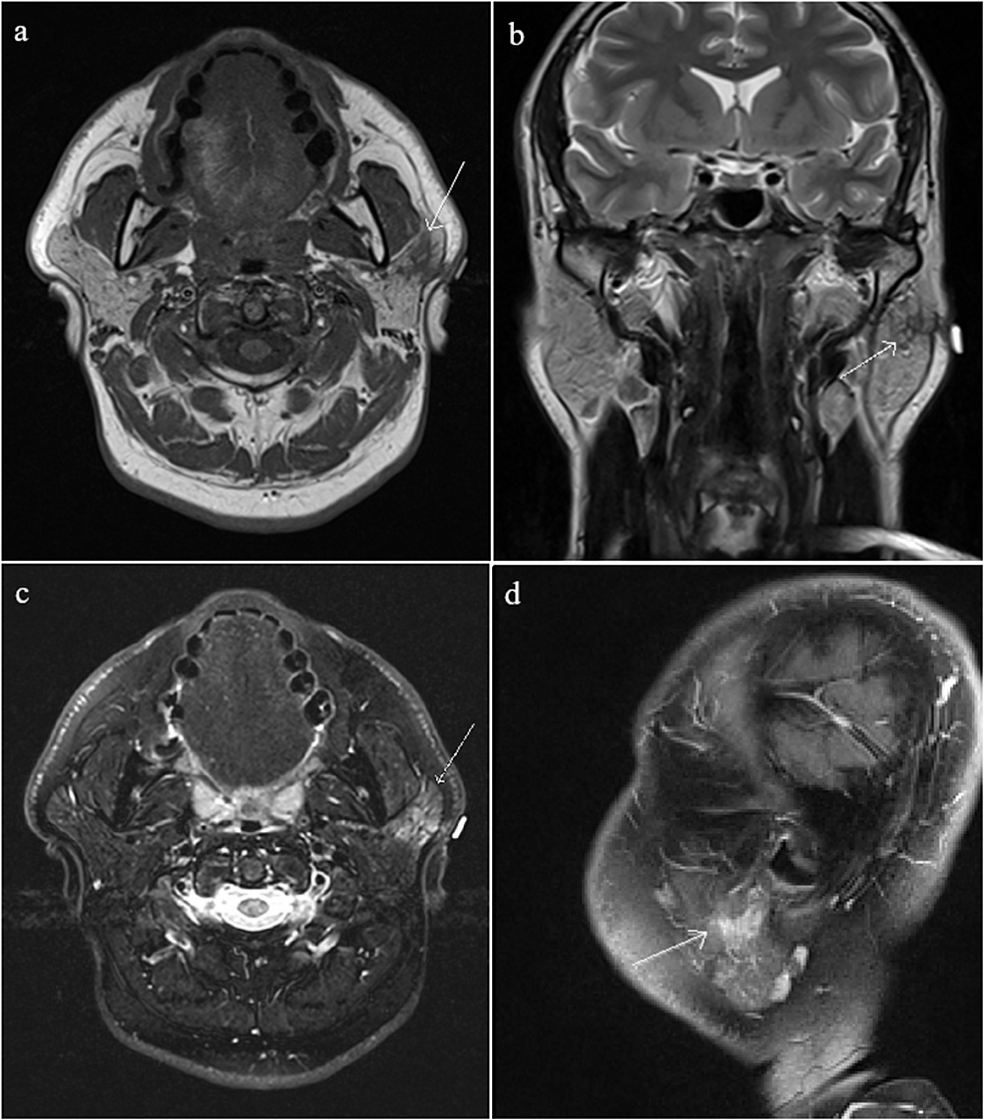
(a) T1-weighted axial and (b) T2-weighted coronal images show an ill-defined linear branching T1 and T2-hypointense area in the area of concern involving the superficial part of the left parotid gland, extending to the subcutaneous soft tissue with adjoining T2-hyperintense areas. (c) Axial short tau inversion recovery (STIR) and (d) sagittal post-contrast T1-weighted images show enhancement along the linear branching area
